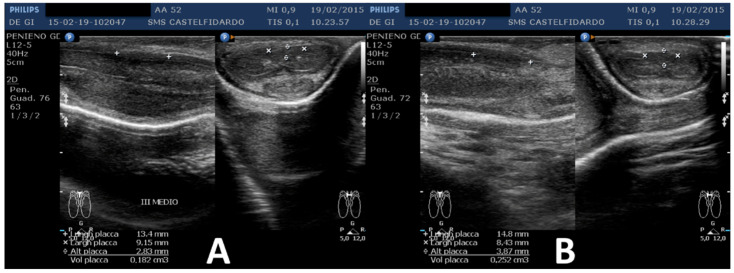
Ultrasonography of the penis and plaque measurement before treatment (longitudinal and transverse views). (A) First plaque, (B) second plaque.
